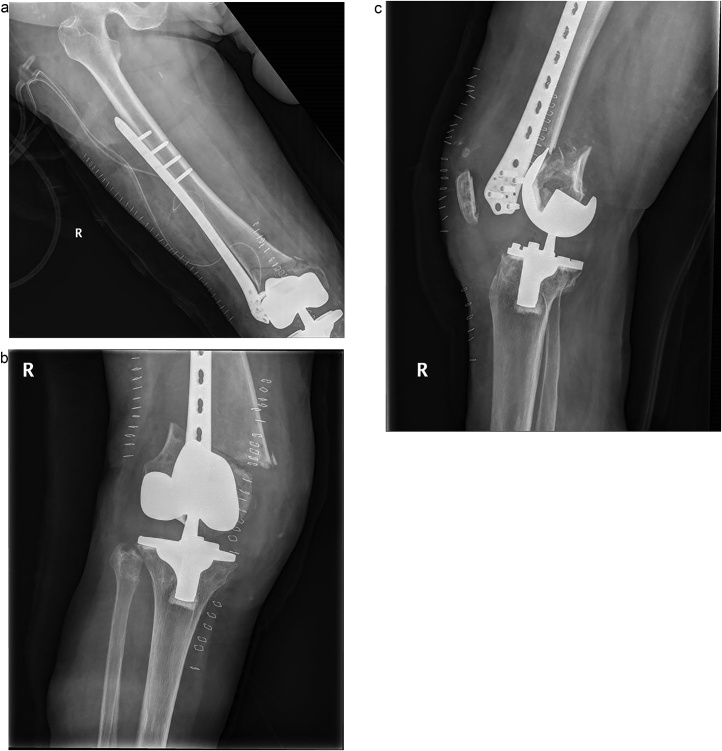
A) Postoperative radiography showed a reduction of displaced fracture and fixaion with LCP on the lateral side of femur (B–C) at postoperative 9th day, radiograph showed the reduction loss of fracture and fixation failure.
